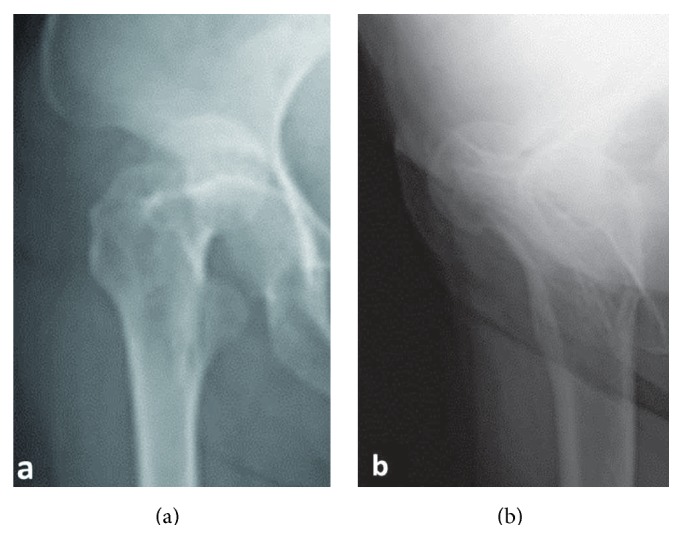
Plain radiographs of the hip joints at the initial visit. (a) Anteroposterior image. (b) Lateral image. These images show anterior dislocation of the right hip joint.
